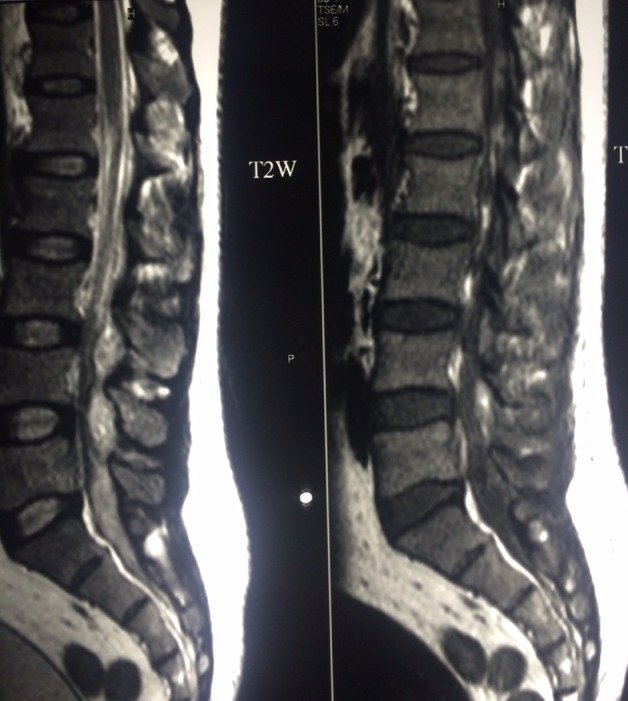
Preoperative MRI sagittal section, T2W (left picture), T1W (right picture) show localized collection of pus in the canal at the level of L3–4 with compression on the dural sac.
